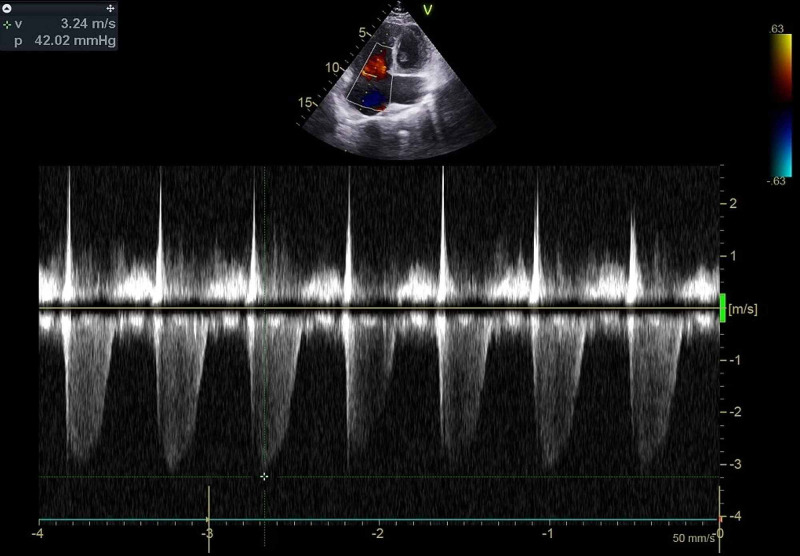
Continuous wave Doppler signal of the tricuspid regurgitation jet
